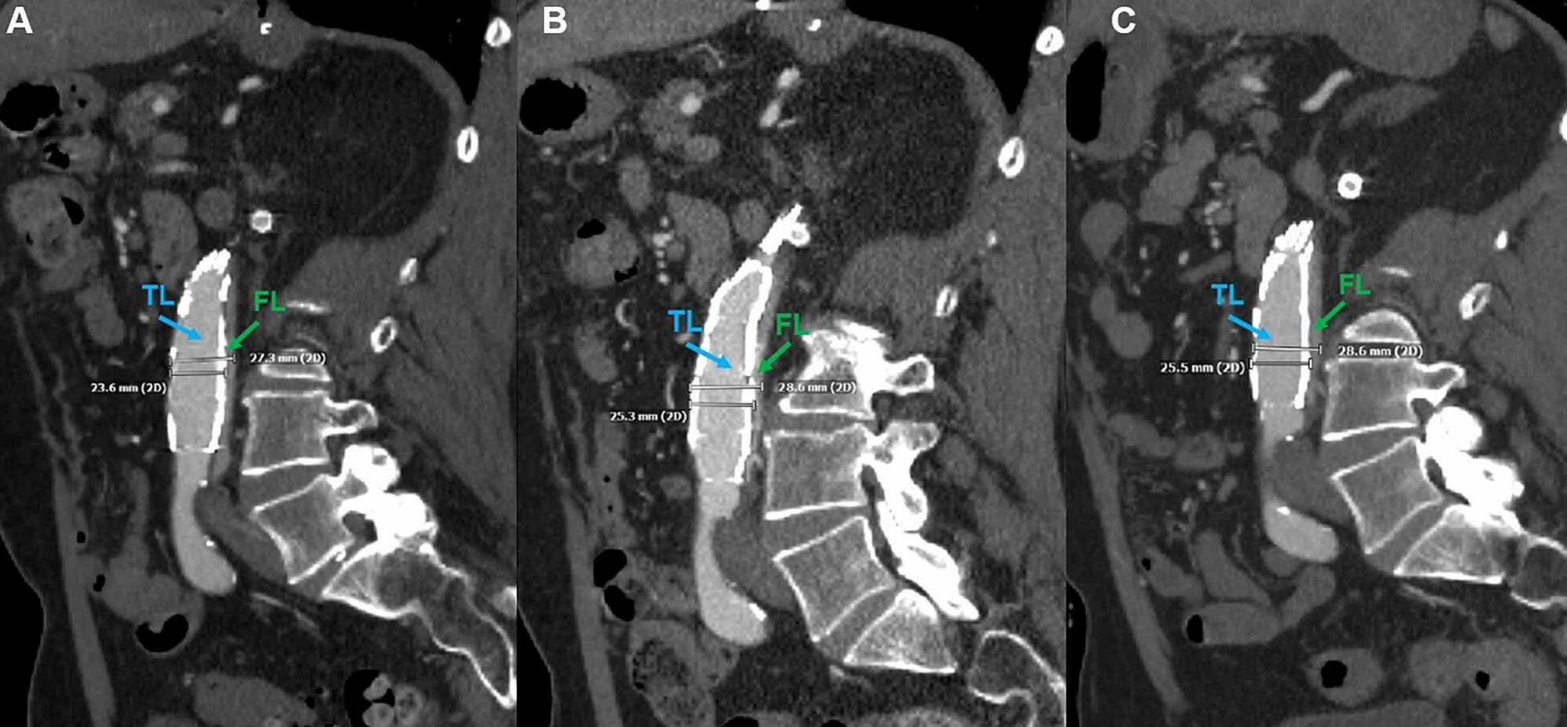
Post-Procedural Short and Mid-Term Surveillance A one month post-procedural sagittal computed tomography angiogram shows the bare metal/open cell stent within the true lumen (blue arrow), excluding the false lumen (green arrow), and terminating appropriately just above the aortic bifurcation (A). The true lumen is noticeably larger in diameter than the false lumen, measuring 23.6 millimeters. A four-month post-procedural sagittal computed tomography angiogram of the same patient shows the bare metal/open cell stent within the true lumen (blue arrow), continuing to exclude the false lumen (green arrow), and terminating appropriately just above the aortic bifurcation (B). The expansile capability of nitinol within the open cell/bare metal stent has increased the true lumen diameter to 25.3 millimeters, while further diminishing the size of the false lumen after four months. A nine-month post-procedural sagittal computed tomography angiogram of the same patient again shows the bare metal/open cell stent within the true lumen (blue arrow), continuing to exclude the false lumen (green arrow), and terminating appropriately just above the aortic bifurcation (C). There is a marginal increase in the true lumen diameter, measuring 25.5 millimeters in comparison to the four-month post-procedural computed tomography angiogram (Figure 7B).
